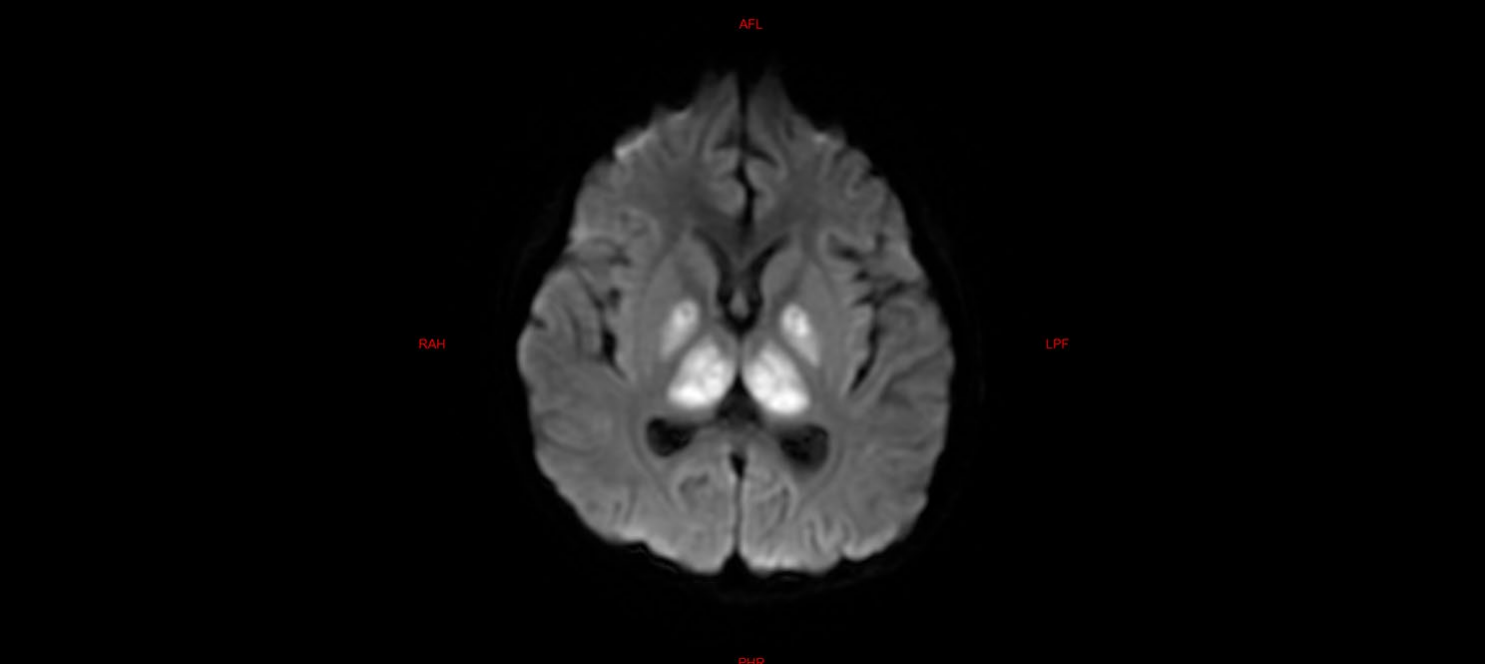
Brain MR DWI sequence of case 5, showing symmetrical diffuse high signal in bilateral basal ganglia and thalamus. The patient was diagnosed with necrotizing encephalopathy. Shortly after admission, there was a rapid deepening of consciousness impairment, leading to cerebral dysfunction, cessation of spontaneous breathing, and concomitant circulatory failure. Ultimately, the patient succumbed to multiple organ failure
